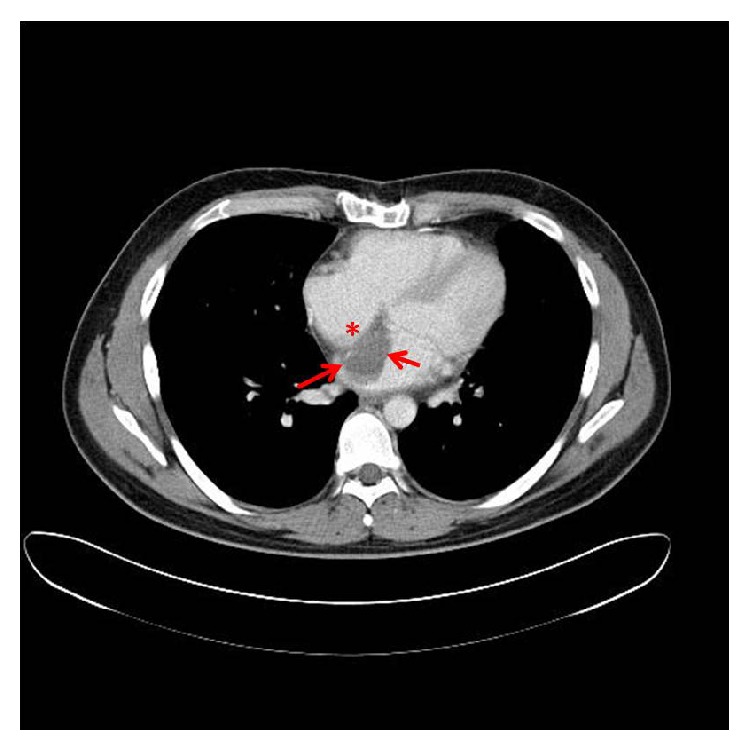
Presentation 3. CT-scan showing tumor of the intra-atrial septum in the left atrium, nongated scan.
